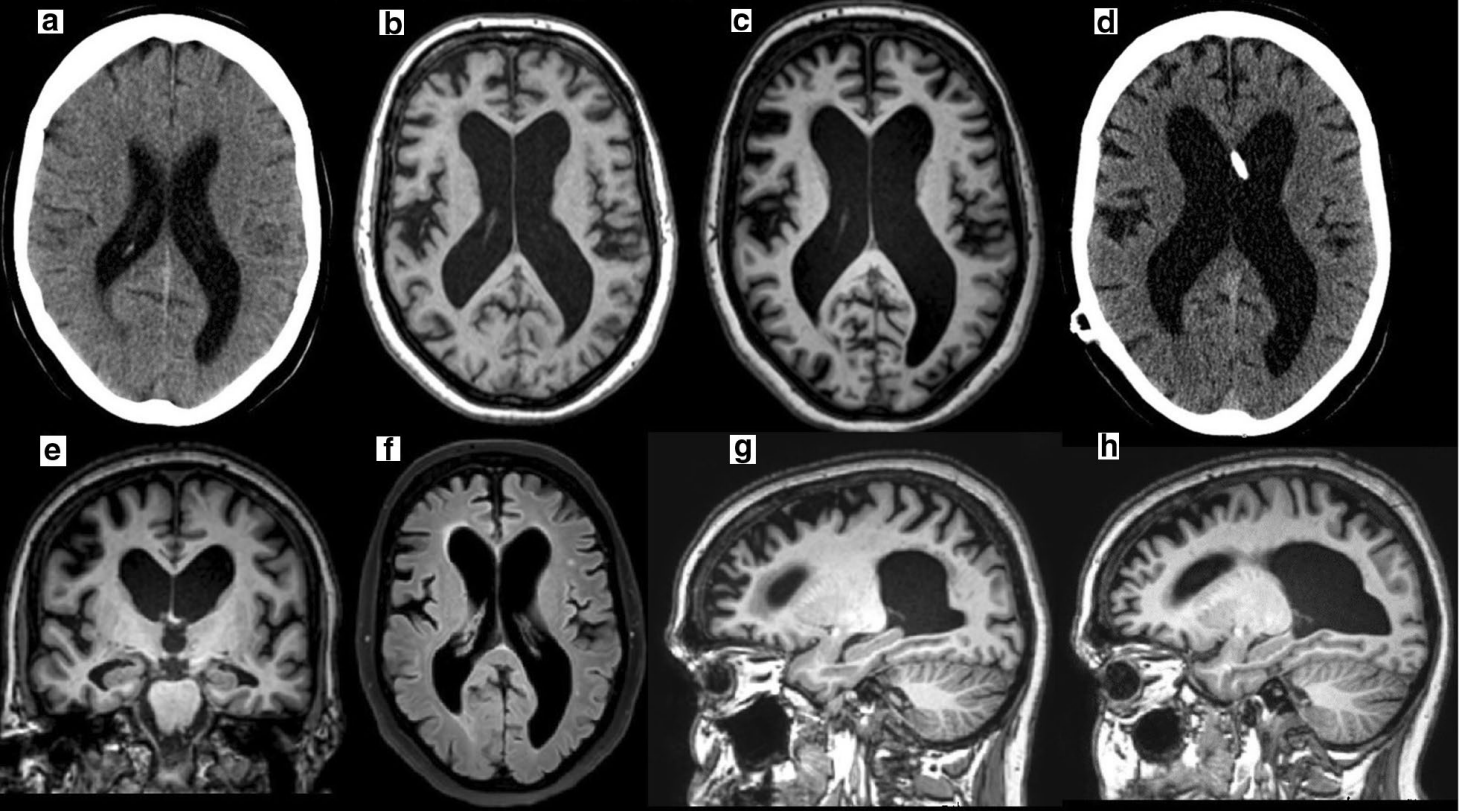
a A brain CT scan that was performed because of an episode of syncope 5 years before the onset of symptoms shows no atrophy (Evans’ index 0.33), but some asymmetry of the occipital horns of the lateral ventricles is apparent. b The first T1weighed MRI, which was performed 1 year after the onset of symptoms, shows the development of frontal cortical atrophy and central atrophy (Evans’ index 0.42) c with some progression over the follow-up time of 1 year (Evans’ index 0.45). d The CT control scan that was performed 6 months after shunt placement shows stable ventricular size (Evans’ index 0.45). e The coronal slice shows hippocampal atrophy (Scheltens grade 2/4) but no disproportionate obliteration of the parasagittal sulci. f The FLAIR sequence shows only minor changes in the white matter. The parasagittal images show that frontal cortical atrophy is more prominent on the *right* (g) compared to the *left side* (h)
